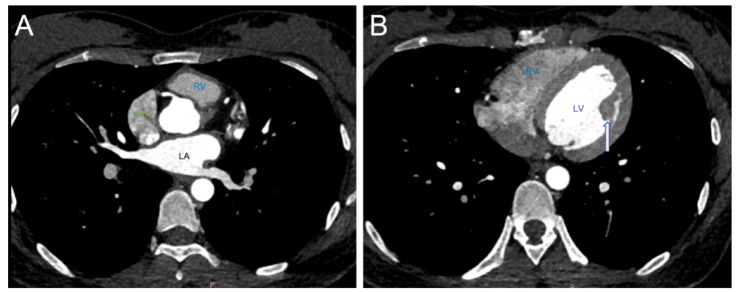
The patient underwent a follow-up cardiac CT scan (A,B), which found no abnormalities in either atrium. The left atrium (LA), right atrium (RA), and right ventricle (RV) were not found. (B). A large papillary muscle in the left ventricle (white arrow), right ventricle (RV), and left ventricle (LV).
